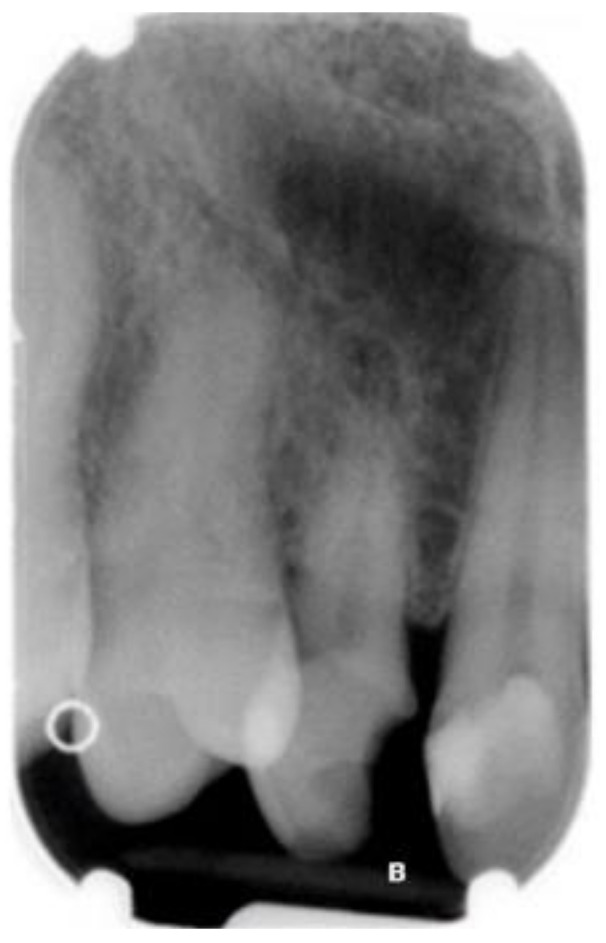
Endoral X-ray performed four months after cuspid extraction to assess the bone healing.
